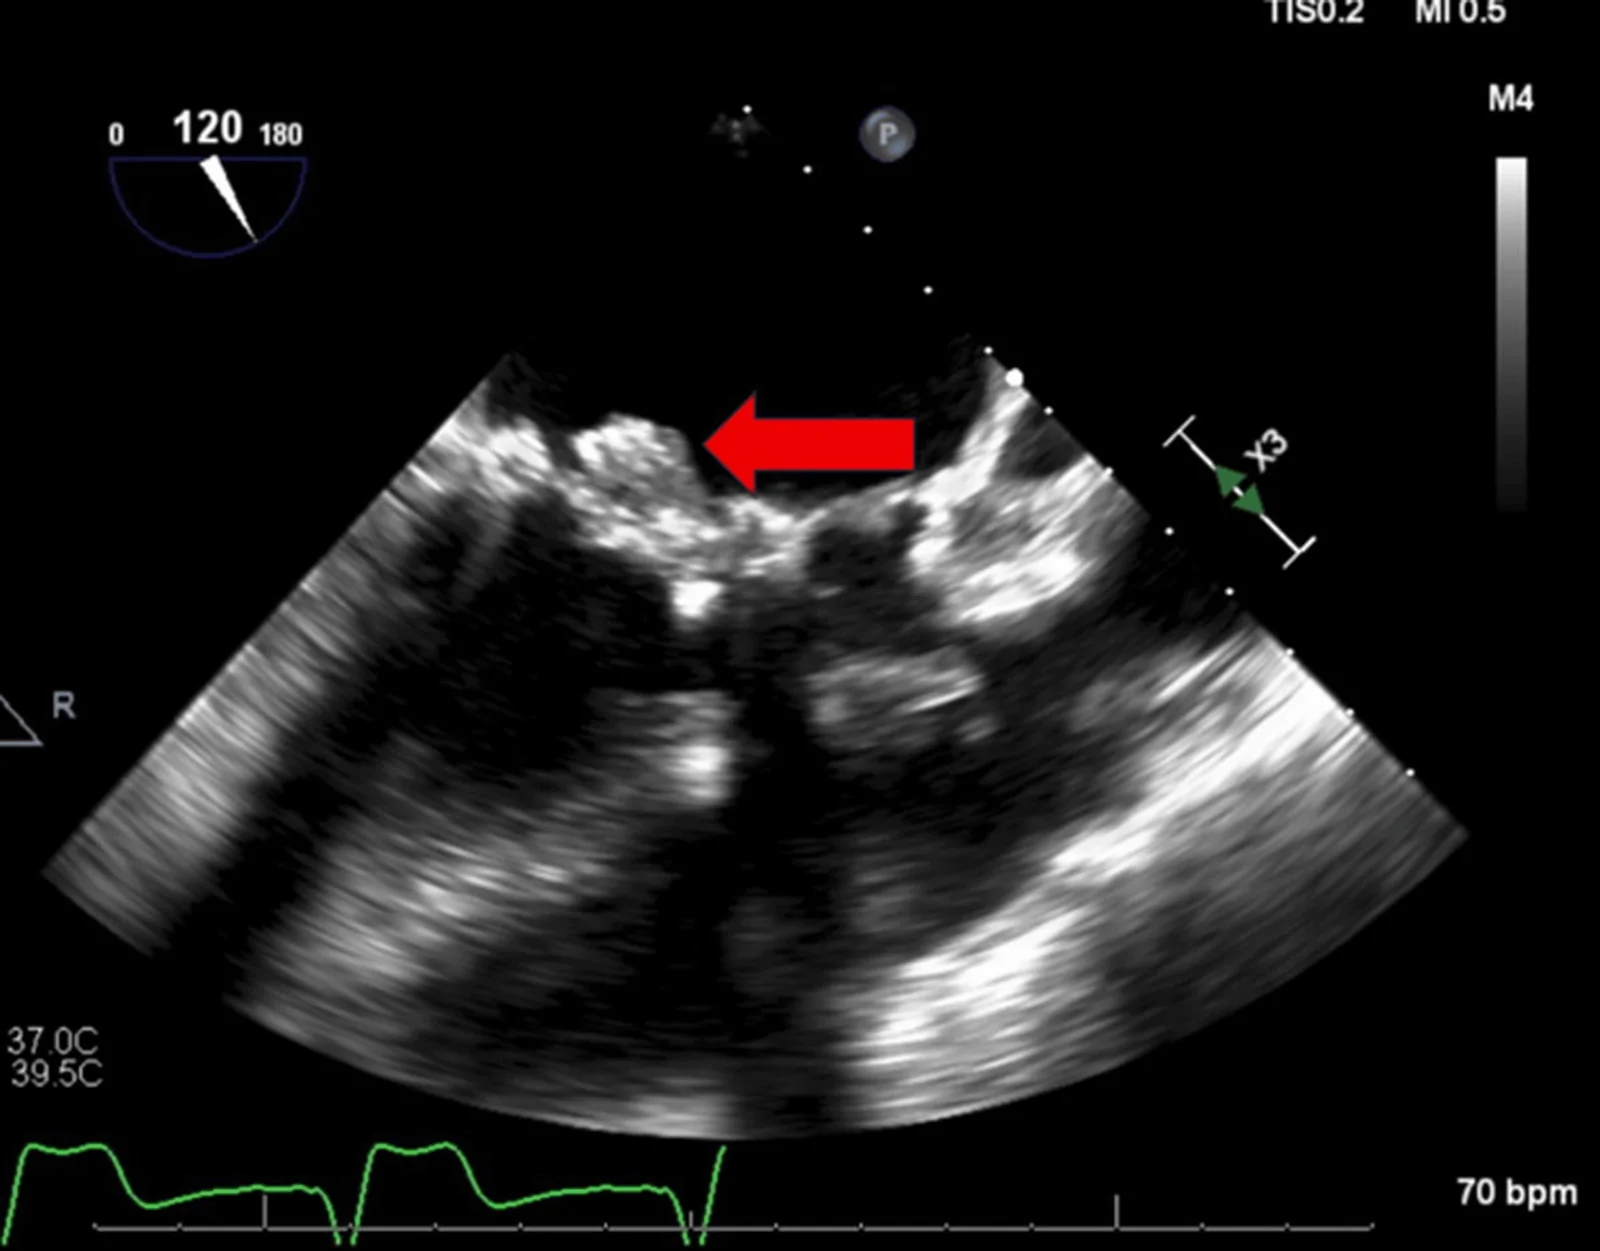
Follow-up transesophageal echocardiogram (TEE), mid-esophageal four-chamber view, performed 14 days after the initial study, demonstrating interval enlargement of a multilobulated, mobile density on a bioprosthetic mitral valve, increasing from ~10 mm to ~16 mm (red arrow)
